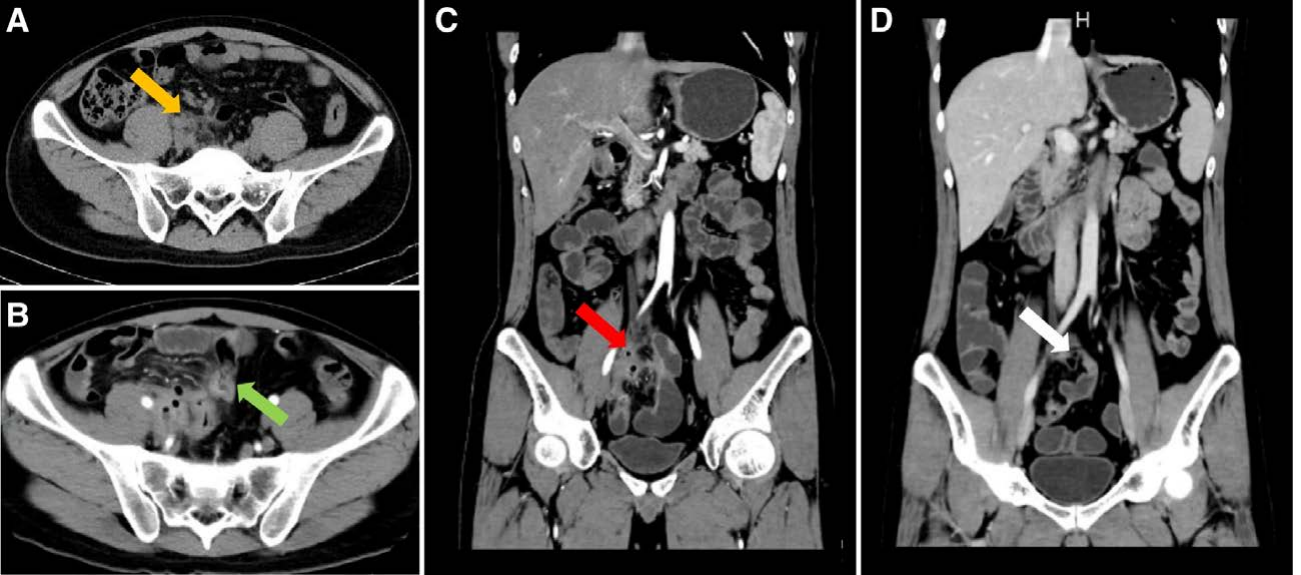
Relevant imaging data during the course of the disease. Abdominal CT scan shows infectious exudation around the mid-ureter (A; orange arrow); contrast-enhanced CT scan shows multiple segmental wall thickening in the small bowel, especially in the ileum, with partial stenosis (B; green arrow). CTE reveals focal small bowel areas of wall thickening and an intestinal fistula (C; red arrow). Reevaluated CTE after 10 weeks of exclusive enteral nutrition reveals absorbed infected lesions and a remaining intestinal fistula (D; white arrow).
